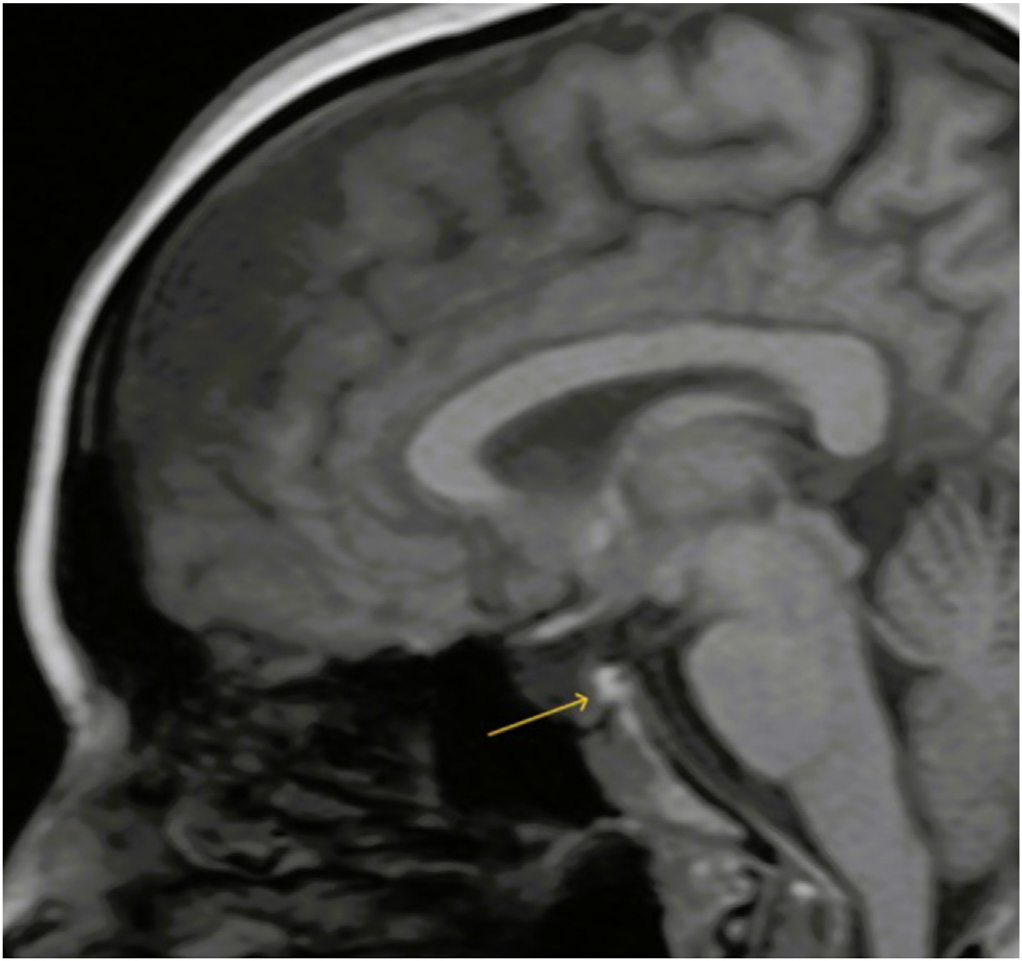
Sagittal T1-weighted MRI of the pituitary gland showing a characteristic hyperintense signal in the posterior pituitary (yellow arrow), indicative of preserved arginine vasopressin function. *MRI* = magnetic resonance imaging.
